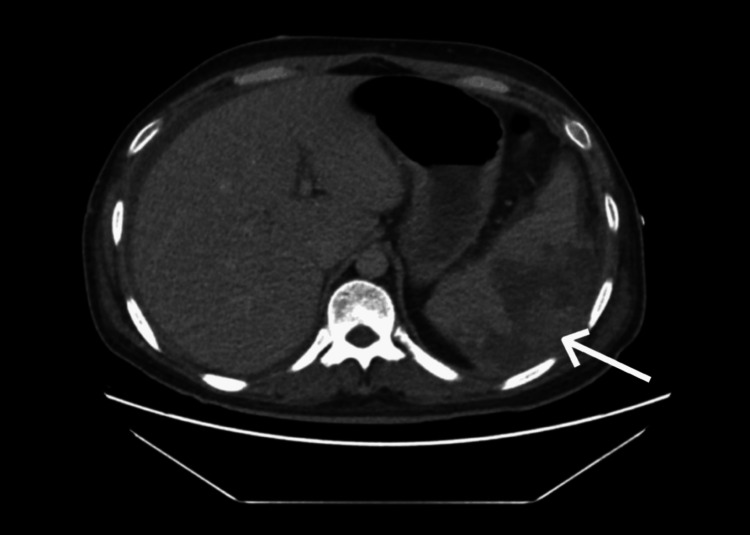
Contrast-enhanced CT scan, axial view of the abdomen, showing splenic abscess rupture
